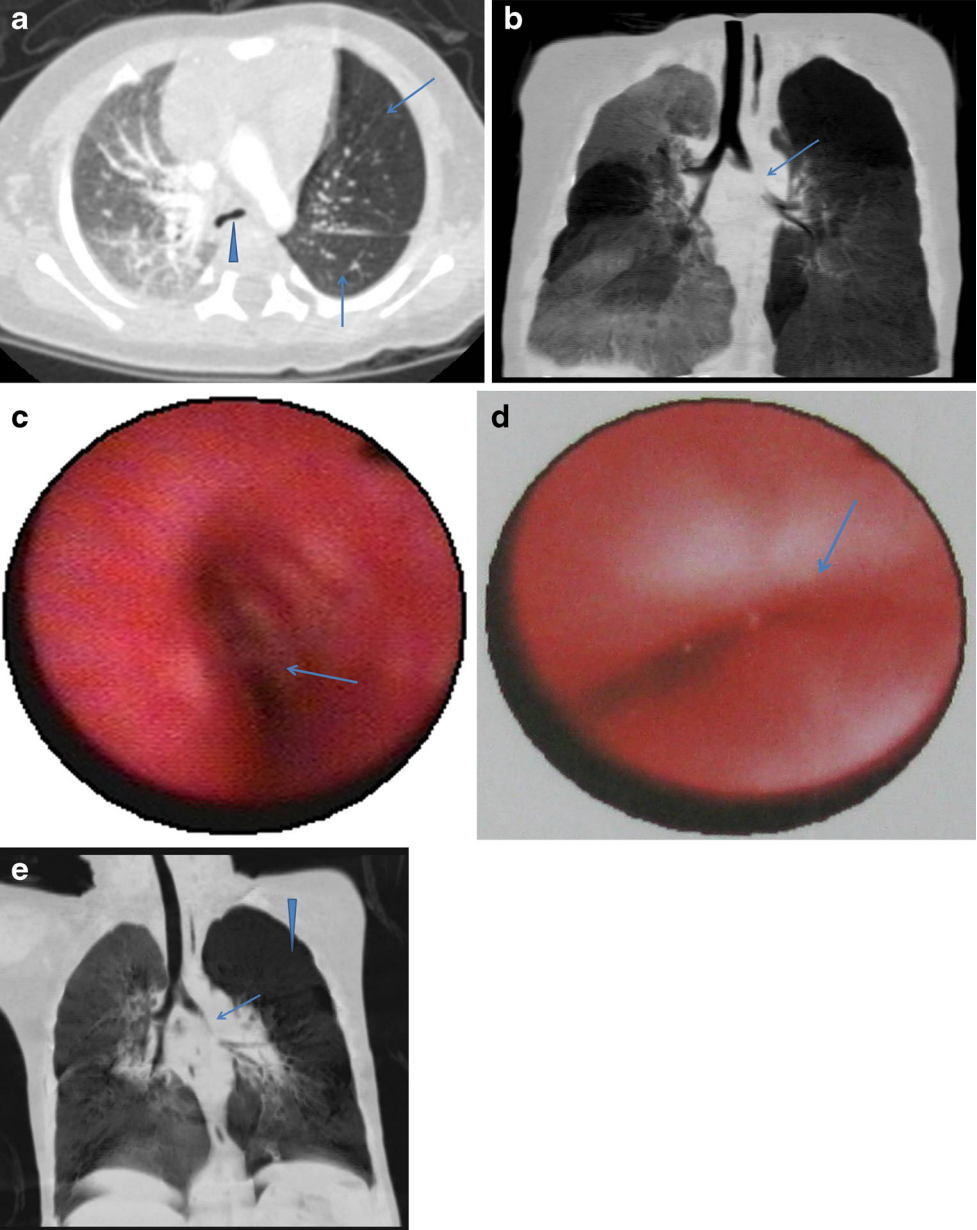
Absent pulmonary valve syndrome with tetralogy of Fallot in a 4-month-old boy. a Multi-slice CT axial and (b) coronal minimum-intensity projection CT images demonstrate carina (*arrowhead* in a) and left main bronchial compression (*arrow* in b). Note the obstructive emphysema in the left lung and right middle lobe (*arrows* in a). c, d Bronchoscopy demonstrates carina compression (*arrow* in c) and left main bronchus bronchomalacia (*arrow* in d). e Multi-slice CT coronal minimum-intensity projection reconstruction 3 months after surgery in the same child, take picture from different angle demonstrates an improved appearance of the left bronchus (*arrow*) and considerable improvement in the right middle lobe and left lung obstructive emphysema (*arrowhead*)
